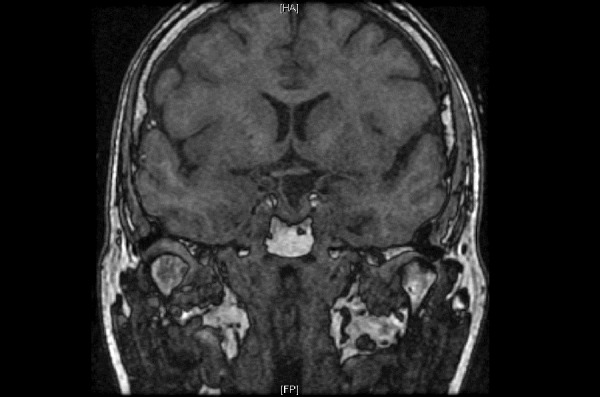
Postoperative coronal T1 MRI shows gross total resection of the tumor
